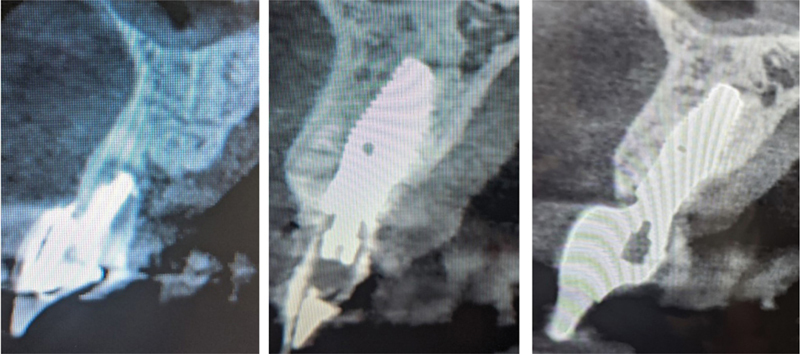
Cone-beam computed tomography (CBCT) cross-sections at the preoperative of the failing tooth (left), immediately following immediate implant placement, and provisional restoration placement (middle) and following 12 months' loading and final restoration placement (right).
